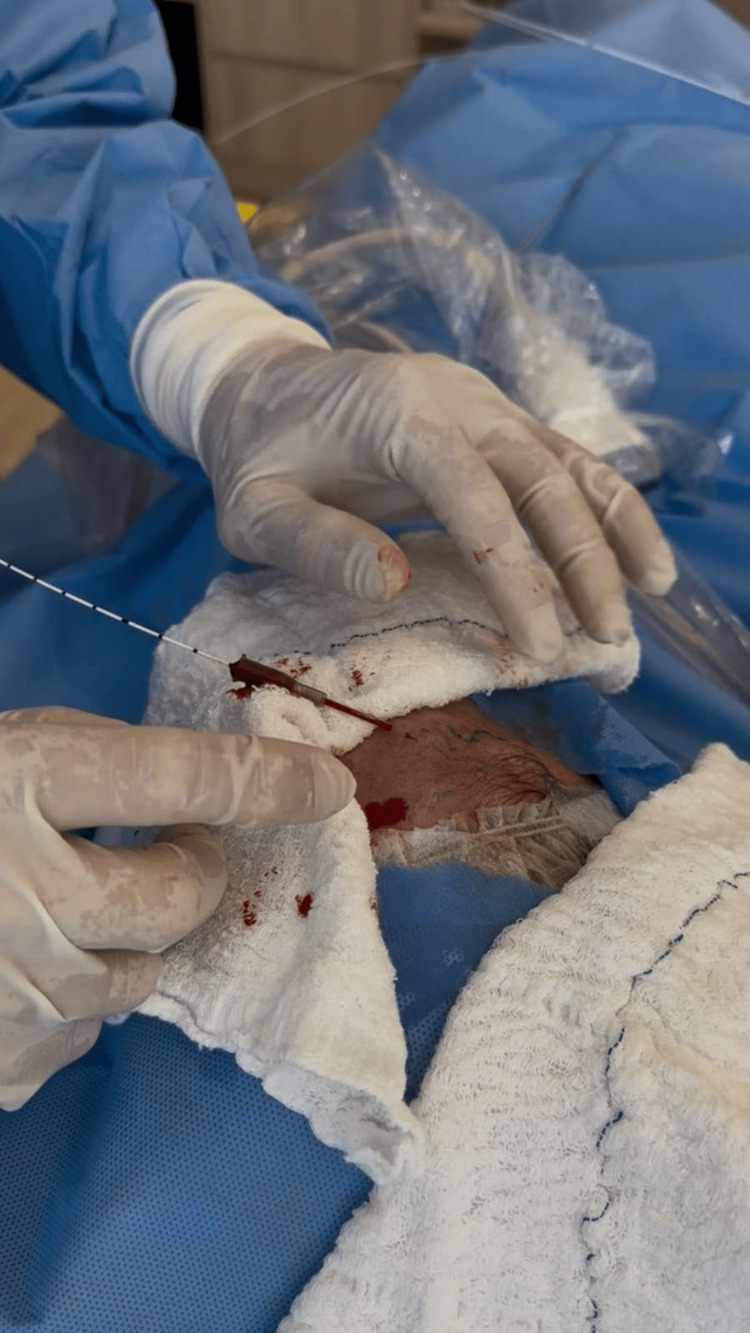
Cannulation of the frontal branch of the superficial temporal artery with advancement of a 400 µm radial fiber within the arterial lumen under ultrasound guidance.
